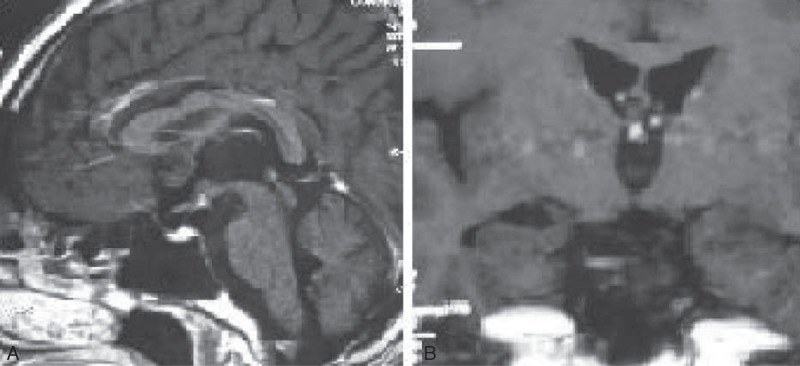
Latest MRI of the patient (almost 2 years after the treatment was given). MRI showed that the abnormality in the hypothalamus on MRI has markedly improved. MRI = magnetic resonance imaging.
